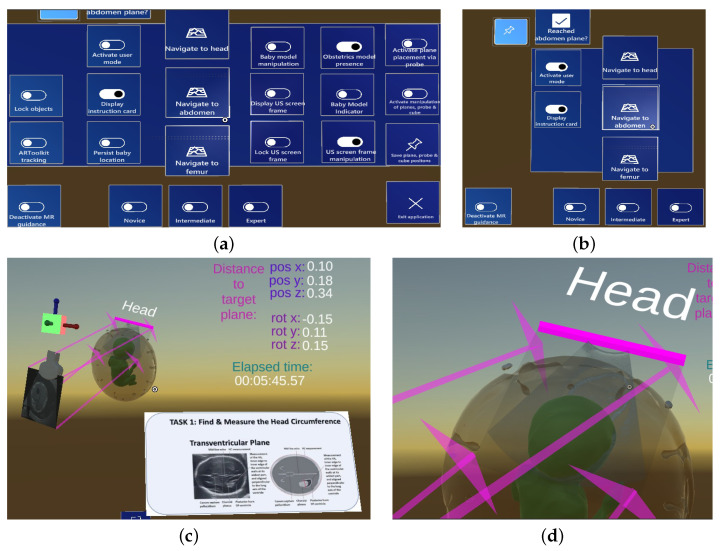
Unity scene showing the various components of the holographic setup: (a) complete holographic menu for experts; (b) reduced holographic menu for the trainee; (c) navigation to head standard plane including the navigation instruction card, navigation arrows, position and rotation offset between probe and target plane and elapsed time for the navigation task; and (d) closeup of the head ultrasound plane including the four pink guidance arrows pointing at the plane’s corners.
